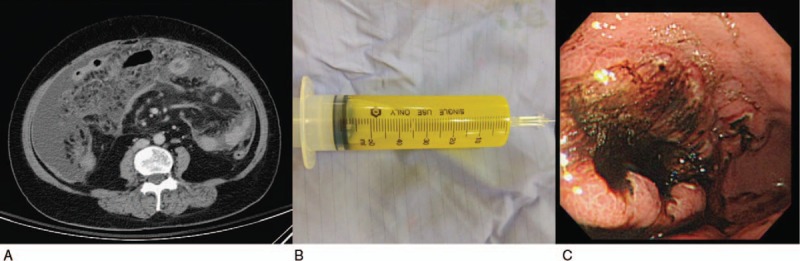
Abdominal computed tomography and esophagogastroduodenoscopy images showing ascites. (A) Diffuse lymphomatous infiltration of the omentum, causing peritoneum thickening. (B) Straw-colored ascites, without blood or chyle. (C) Infiltration of ulcerative gastric mass with coffee-ground material.
